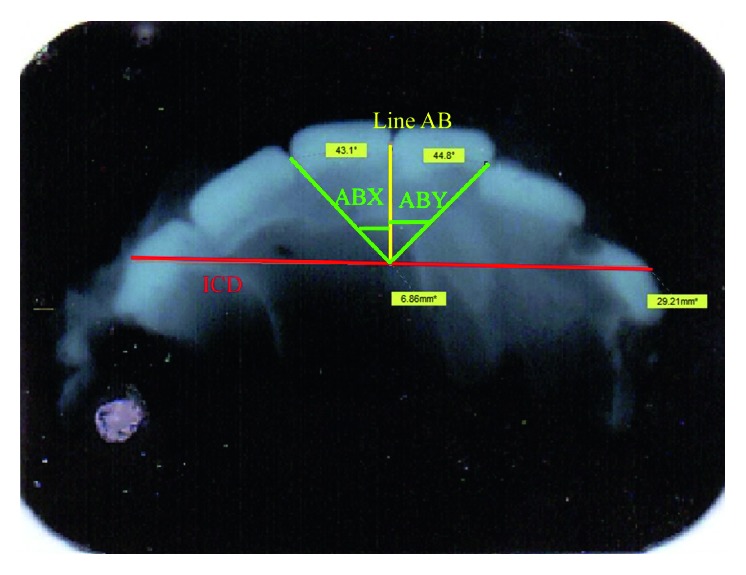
Measurement of ICD, line AB, and angles ABY and ABX radiographically using Sidexis Next Generation software.
